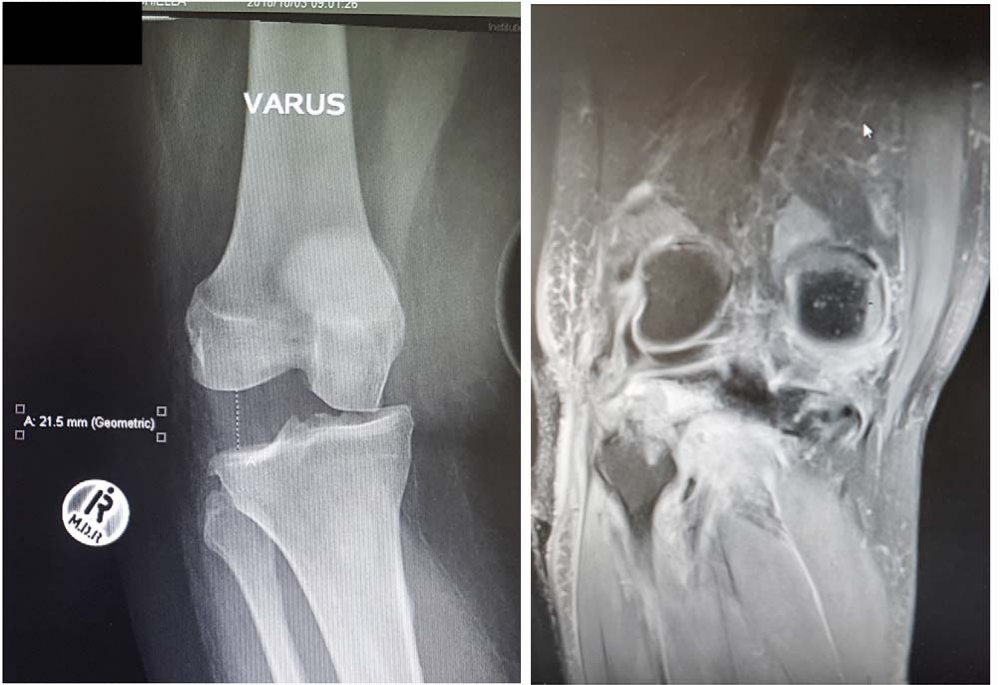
Imaging in a patient with a suspected MLKI. Left: AP varus stress radiograph shows a large lateral joint gap indicative of a complete disruption of the LCL, associated disruption of cruciate ligaments should be suspected. Right: The injury can also be seen on coronal T2 MRI with disruption of the posterolateral complex.
